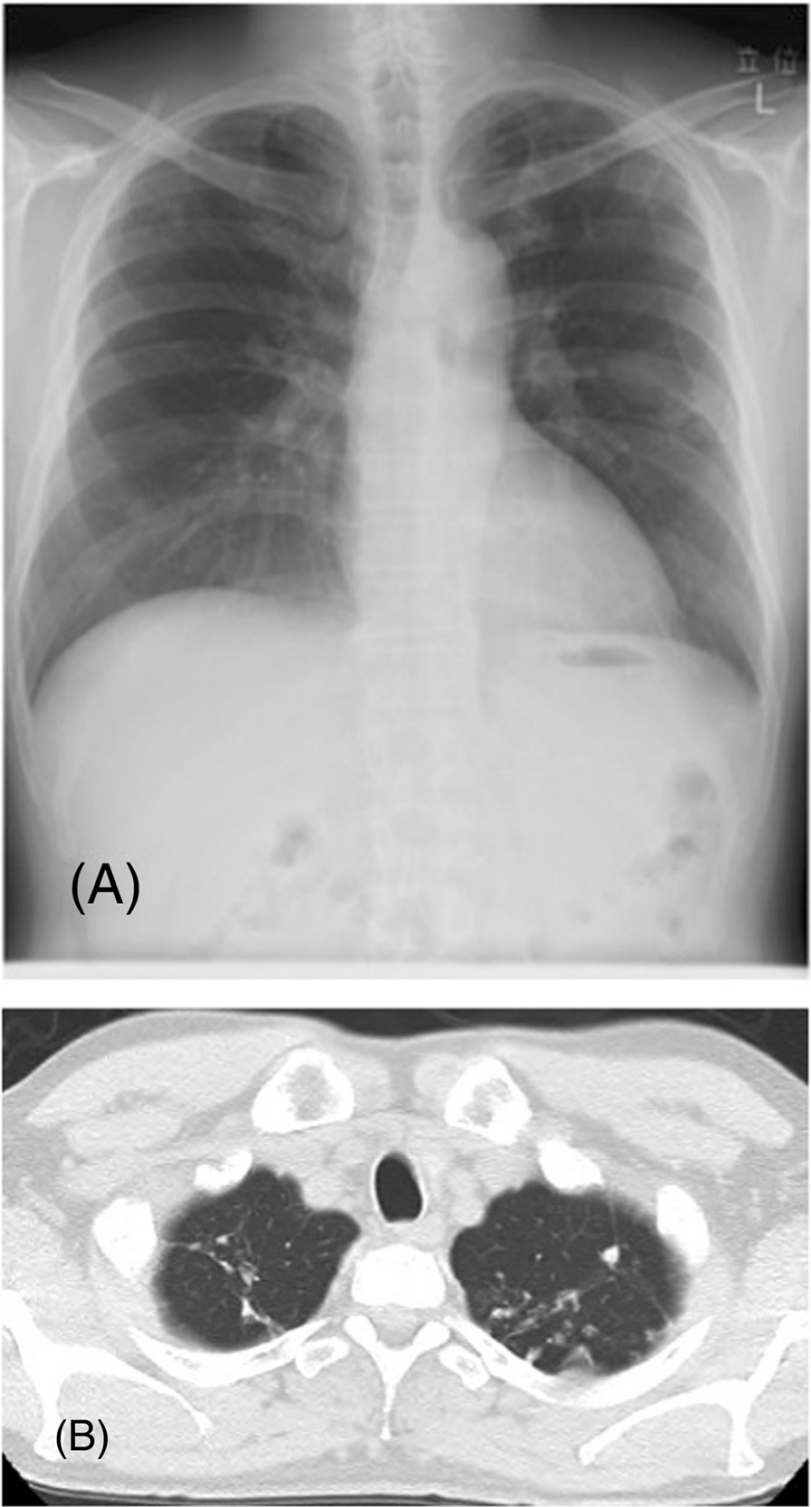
Chest X-ray and CT after 12 months of treatment. Chest X-ray after 12 months of treatment (A) revealed a decrease in the pleural effusion. Chest CT (B) showed that the multifocal nodules tended to disappear.
